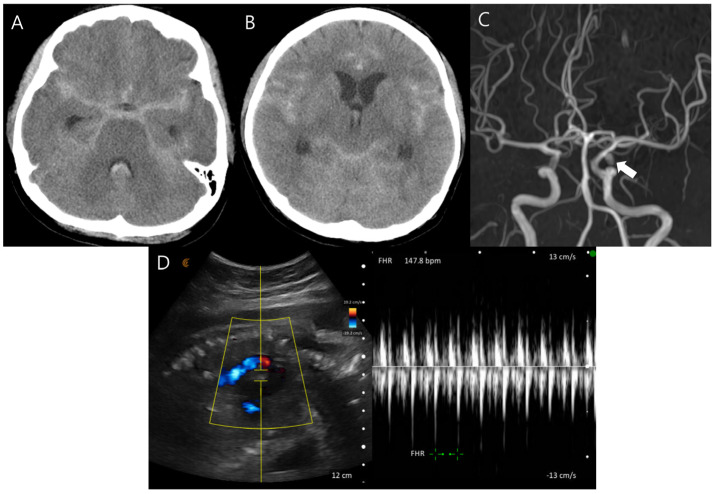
(A,B) Initial computed tomography (CT) shows diffuse subarachnoid hemorrhage with significant obstructive hydrocephalus. (C) Magnetic resonance angiography demonstrates a 5 mm-sized aneurysm at the left posterior communicating artery (white arrow). (D) Obstetric ultrasonography shows a stable fetal heart rate. The yellow area represents the sample region used for Doppler flow analysis.
